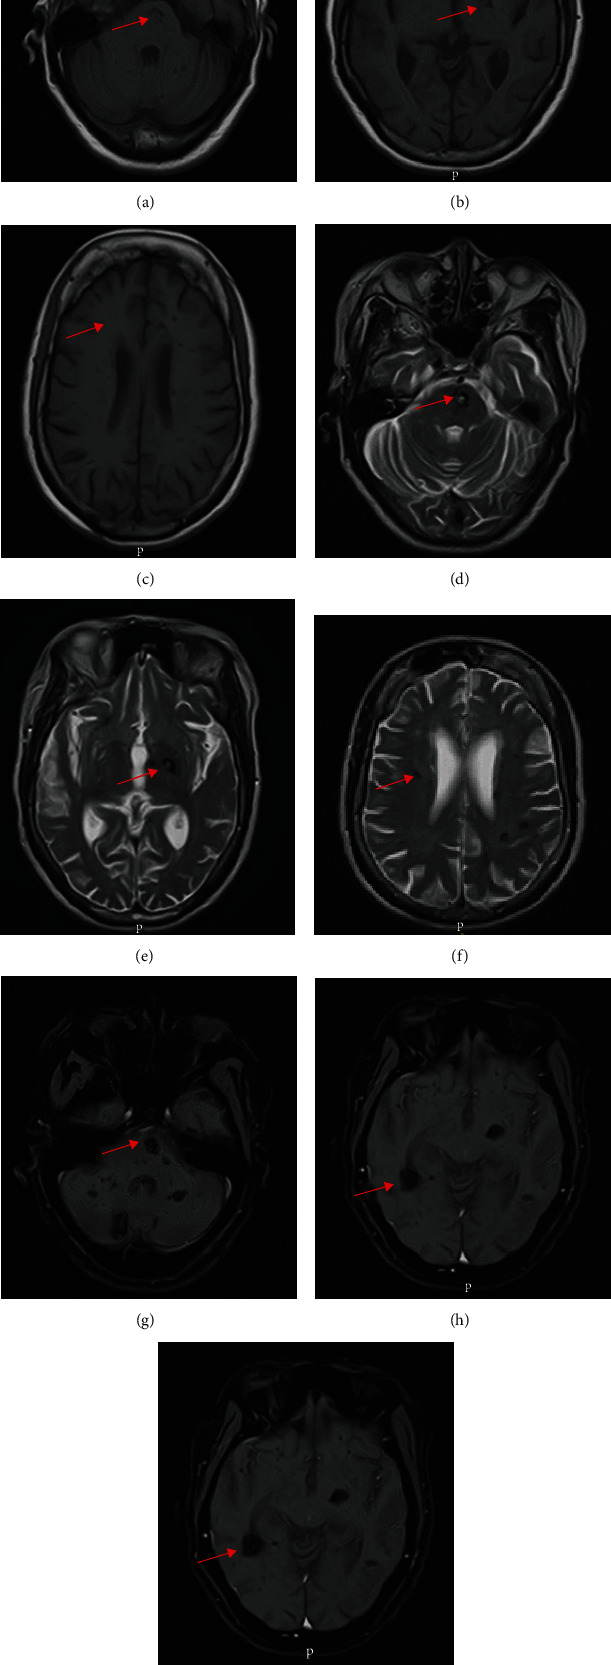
Axial brain MRI: (a–c) T1W, (d–f) T2W, and (g–i) T1W postgadolinium enhanced images showing multiple diffuse cerebellar, pons, bilateral basal ganglia, and cerebral lesions of varying sizes (red arrows); the larger lesions on T1W and T2W images showed hyperintense core and peripheral rim of hypointensity due to hemosiderin in keeping with multiple cerebral cavernomas. No enhancement was noted postgadolinium contrast administration (g–i).
